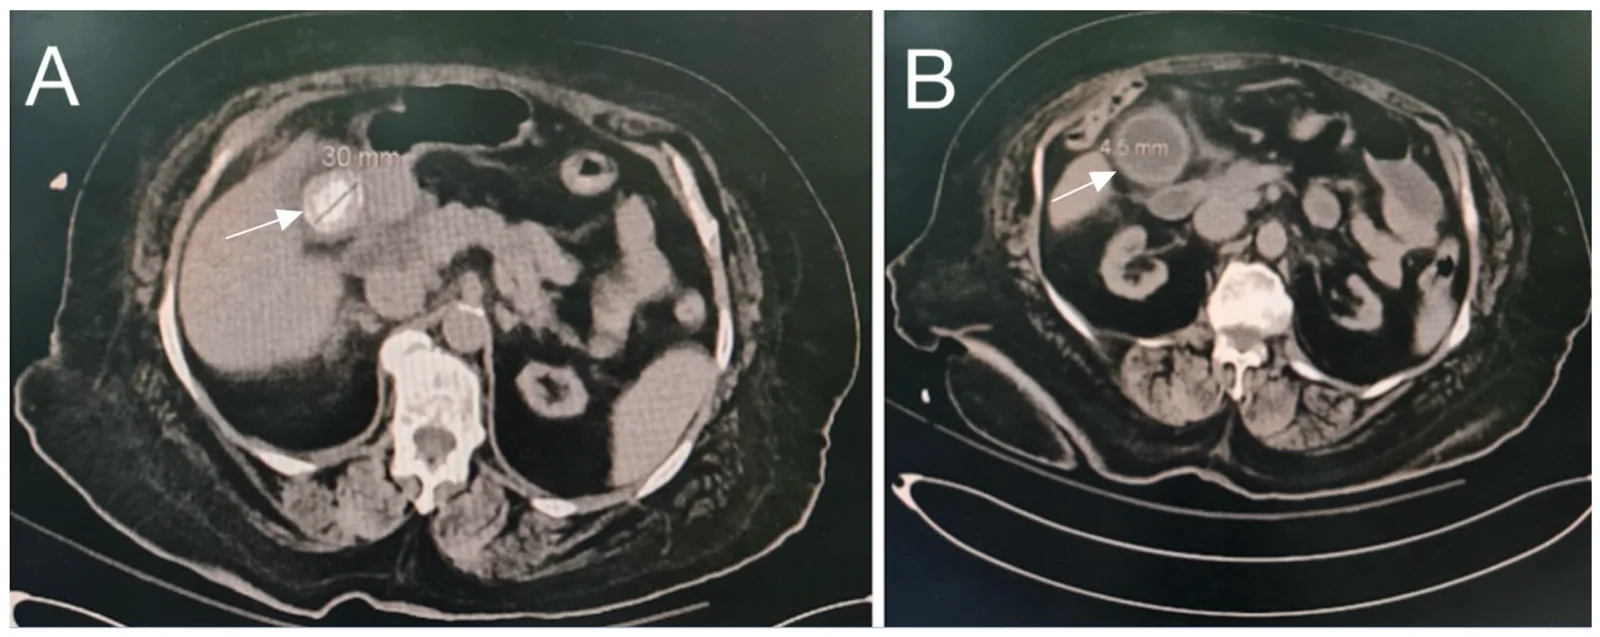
CT scan of the abdomen and pelvis: (A) the gallbladder demonstrates an intraluminal hyper-dense stone located in the fundus, measuring 3 cm (arrow). (B) thickening of the gallbladder wall (0.4 cm) with surrounding fat stranding and minimal pericholecystic free fluid, indicative of inflammation (arrow).
